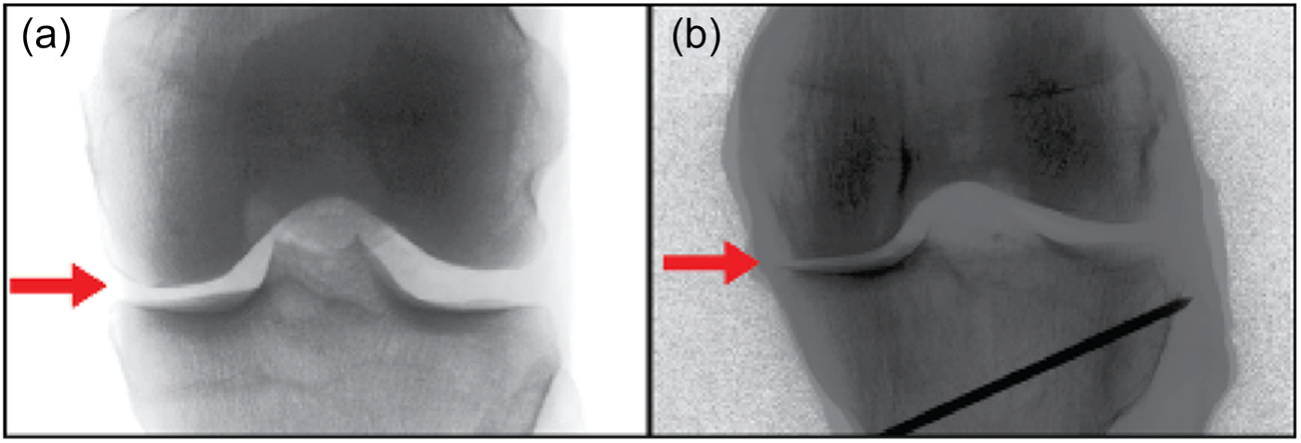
Antero‐posterior radiographic comparison of healthy (a) and arthritic (b) knee. Arthritic knee (b) has medial compartment joint space loss (Kellgren*–*Lawrence grade 2).
